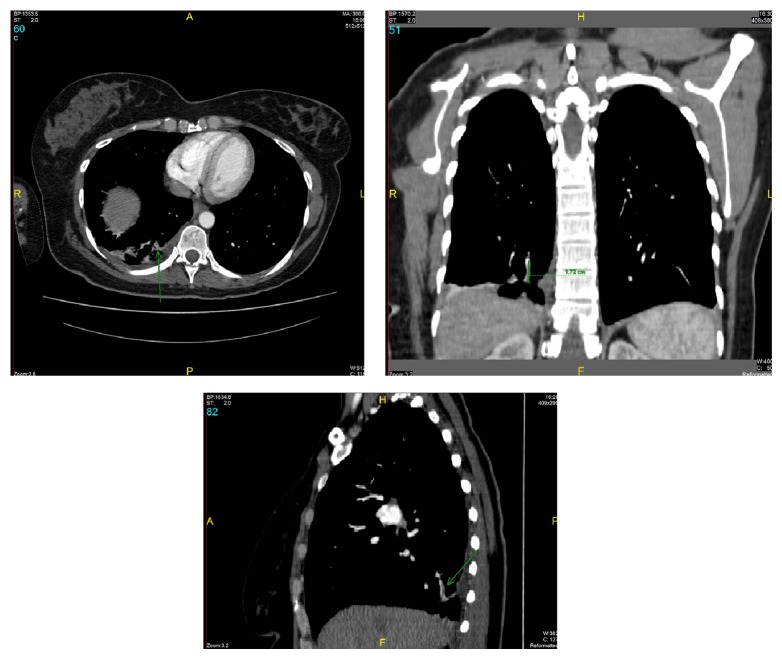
Computed tomography angiogram (CTA) transverse, coronal, and sagittal images depicting the presence of an acute pulmonary embolism (PE) in the subsegmental posterior basal segment artery of the right lower lobe indicated by the green arrows.
